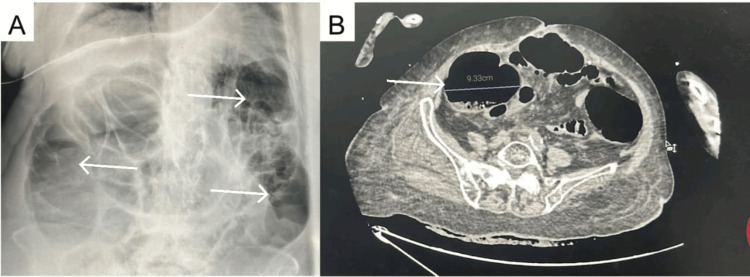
A. Plain abdominal radiograph showing an air-distended colon (arrow) B. CT scan showing a cecal diameter of 9.3 cm (arrow)
